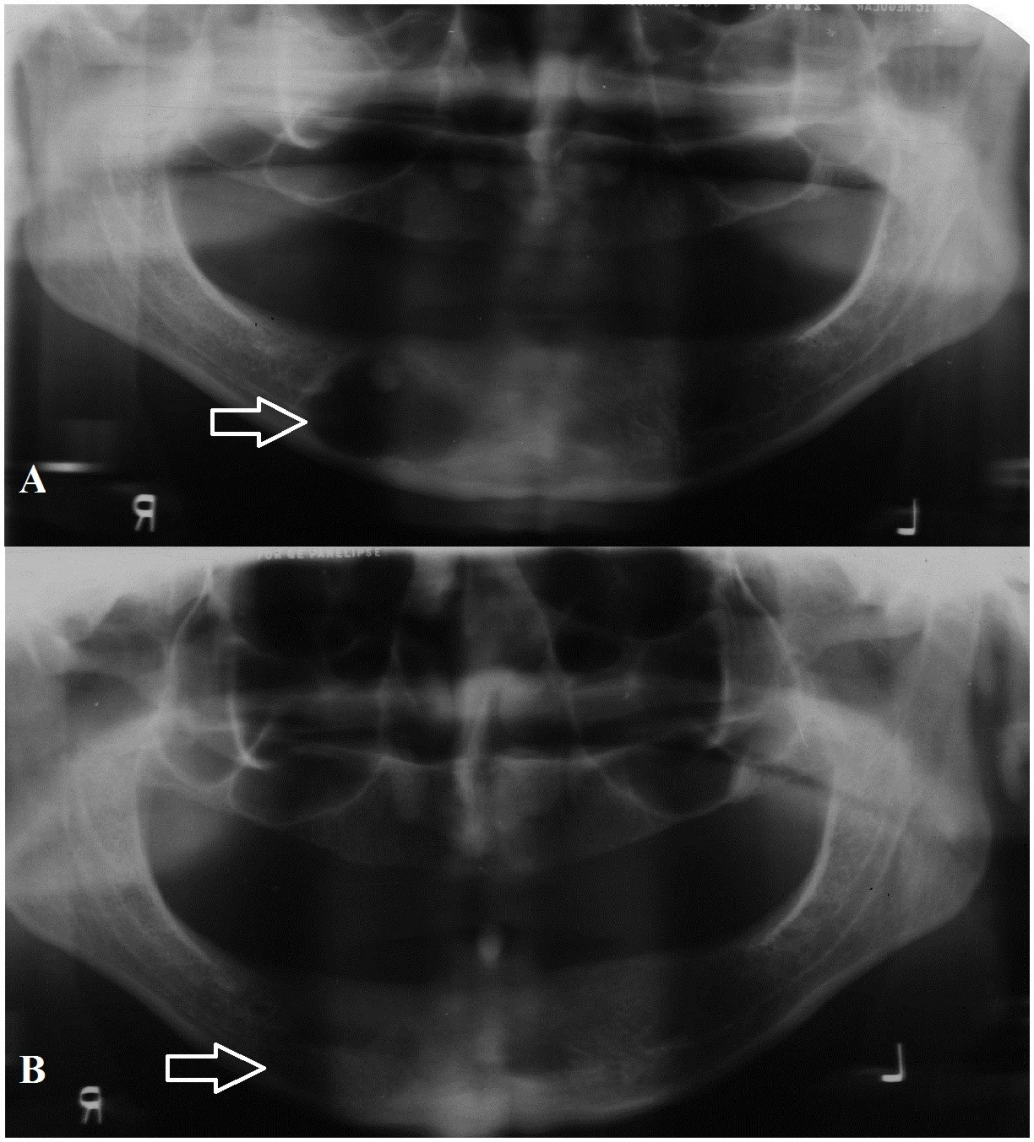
A: Panoramic radiograph showing incidentally found residual cyst in the mandible with calcification. B: Two year post-operative radiograph of same patient showing bone regeneration in the defect.
